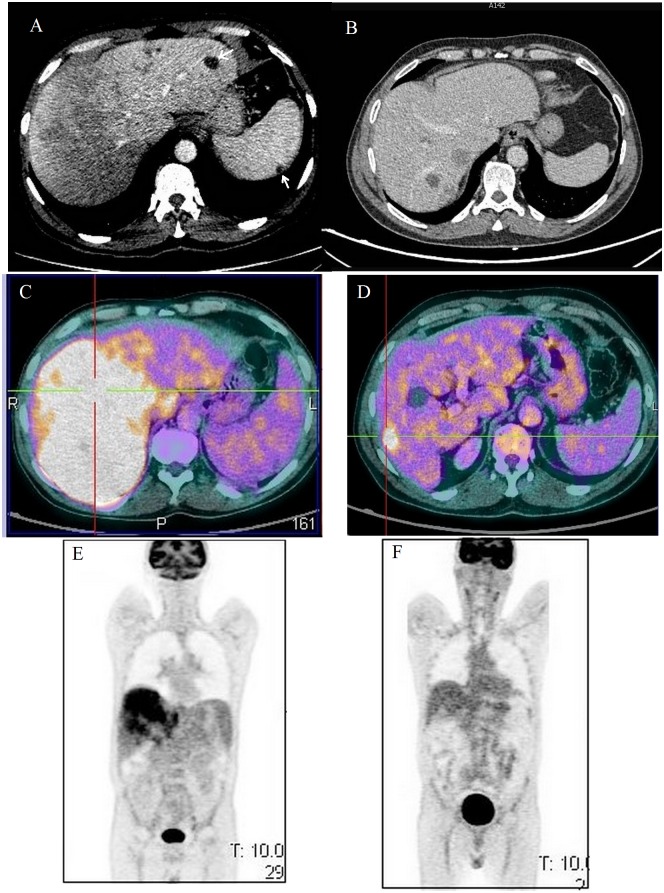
Imaging characteristics and evolution of hepatic toxocariasis. Abdominal computed tomography (CT) scan before (A) and 2 years after treatment (B). Panel A shows diffuse patchy infiltrate of the liver with heterogeneous low-attenuated lesions and cystic lesions (white arrow); Cystic lesion of the spleen (white arrow). Positron emission tomography/computed tomography (PET/CT) before (C) and (E) and 2 years after treatment (D) and (F).
